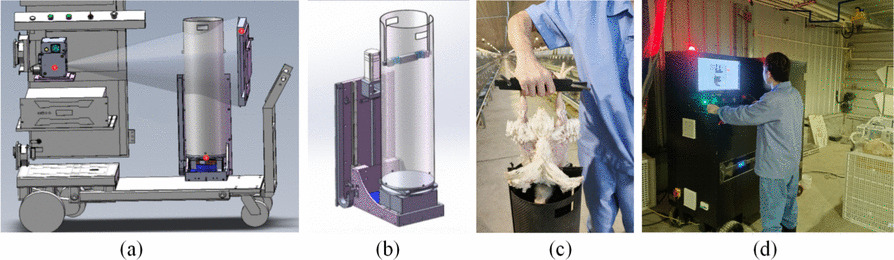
Mobile digital radiography (DR) imaging system for in vivo phenotyping of live chickens. a Overall structure of the mobile DR imaging system, including the X-ray source, detector, and control unit. b Customized sample chamber designed for stable positioning of live chickens during imaging. c Representative posture of a live chicken during in vivo DR image acquisition. d On-farm deployment of the DR system demonstrating high-throughput in vivo image acquisition under practical breeding conditions
